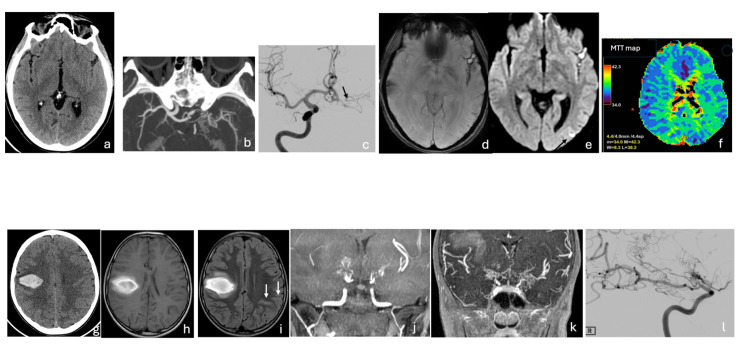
Moyamoya Disease. CT (a,g), CTA-MIP reconstructions (b); DSA (c,l); MRI: SWI (d), DWI (e), T1-FSE (h), FLAIR (i), MRA-TOF (j), MRA-CE (k), DSC-MTT maps (f). (a–f) A 46-year-old patient with right hemiparesis. Axial CT (a) shows obliteration of the left insular subarachnoid spaces. Axial CTA-MIP reconstruction (b) shows occlusion of the terminal left ICA and carotid bifurcation, with absence of the M1 trunk of MCA replaced by a plexiform network. Antero-posterior ICA angiogram (c) shows normal opacification of the right ICA and its branches and confirm left terminal ICA/M1 occlusion with leptomeningeal collaterals from the ACA (black arrow). MRI reveals hemosiderin in the left Sylvian fissure (d) and a small ischemic lesion in temporal lobe (black arrow in (e)). Perfusion map demonstrates delayed MCA flow with MTT asymmetry (f). (g–l) A 9-year-old child presenting with sudden loss of consciousness and left hemiparesis. CT (g) and MRI (T1 in (h), FLAIR in (i)) demonstrate a parenchymal hematoma in the corona radiata; linear FLAIR hyperintensities in the left parietal sulci (white arrows in (i)) represent prominent leptomeningeal collateral vessels (ivy sign). TOF-MRA-MIP (j) and contrast-enhanced MRA (MIP/MPR reconstructions in (k)) show bilateral supraclinoid ICA and M1 occlusion with moyamoya vessels from lenticulostriate arteries. Oblique ICA angiogram (l) confirms bilateral ICA terminus occlusion (black arrow) and M1 replacement by a plexiform collateral network. CT, Computed Tomography; CTA, Computed Tomography Angiography; MIP, Maximum Intensity Projection; MPR, Multi-Planar Reconstruction; DSA, Digital Subtraction Angiogram; MRI, Magnetic Resonance Imaging; SWI, Susceptibility Weighted Imaging; DWI, Diffusion Weighted Imaging; T1-FSE, T1-Weighted Fast Spin Echo; FLAIR, Fluid Attenuated Inversion Recovery; MRA-TOF, Magnetic Resonance Angiography—Time of Flight; MRA-CE, Magnetic Resonance Angiography—Contrast Enhanced; DSC, Dynamic Susceptibility Contrast; MTT, Mean Transit Time; ICA, Internal Carotid Artery; MCA, Middle Cerebral Artery.
